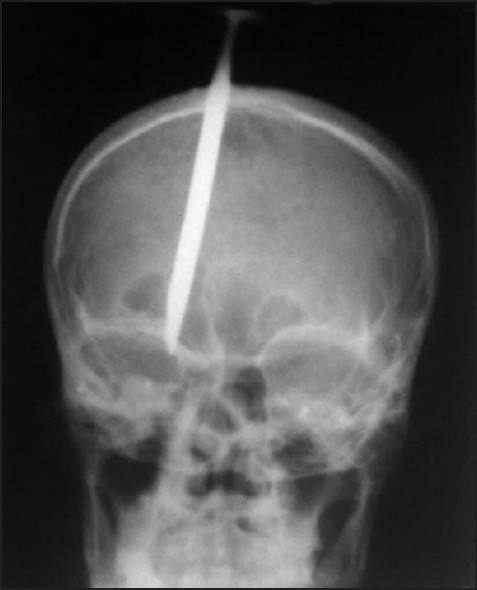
X-ray AP view showing nail *in situ*
